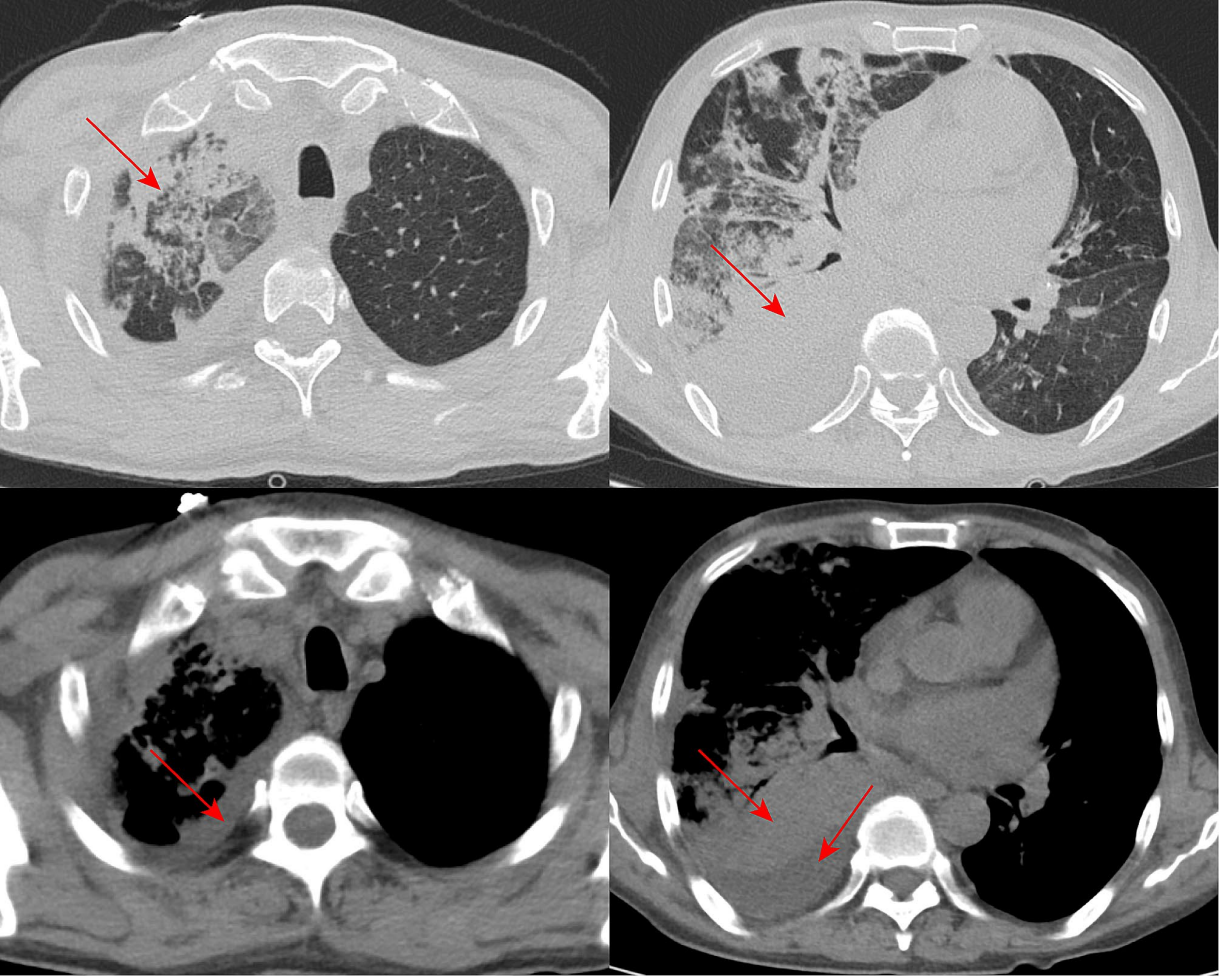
CT chest scan of the patient on admission to the emergency room
